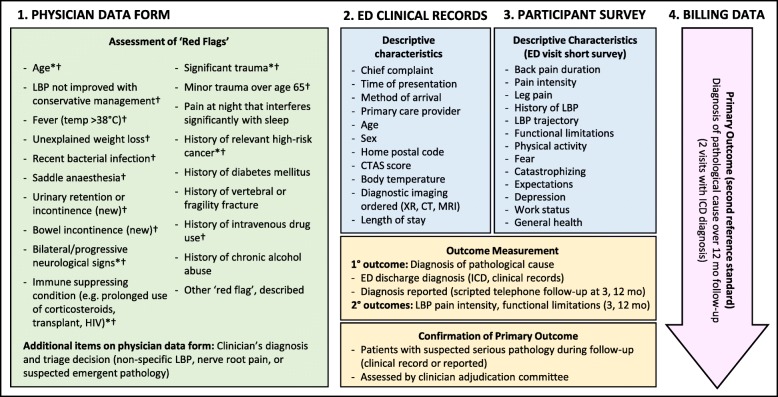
Baseline variables, including red flags and descriptive characteristics, and outcome variables of four data sources. The asterisk indicates the red flag with evidence of positive likelihood ratio > 5.0; the dagger represents the red flag described in the American College of Physicians low back pain clinical practice guideline [9] or by the American College of Radiology [8]; LBP, low back pain; CTAS, Canadian Triage and Acuity Score; ICD, International Classification of Disease; XR, plain-film X-ray; CT, computed tomography; MRI, magnetic resonance imaging; ED, emergency department
